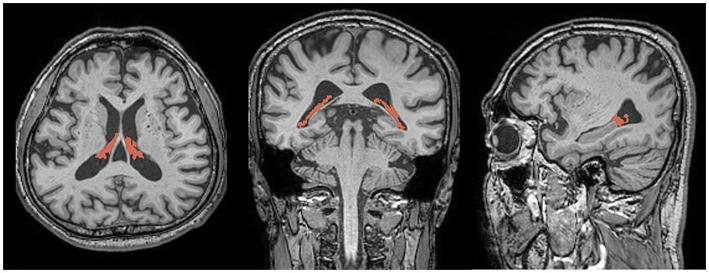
Representative images showing choroid plexus segmentation (red) overlaid on three-dimensional T1-weighted magnetic resonance images in the axial (left), coronal (center), and sagittal (right) planes.
